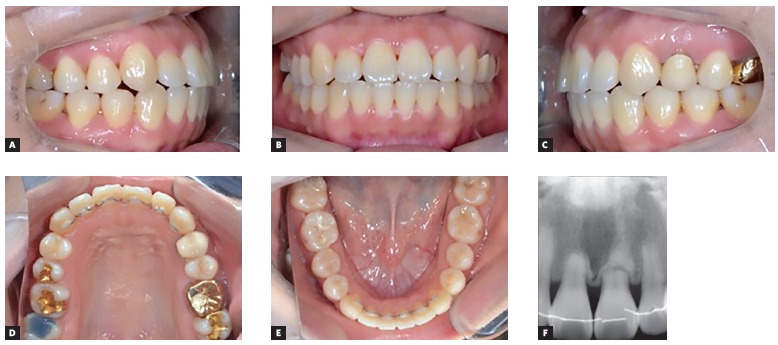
A-E) 3-year follow-up intraoral photographs and F) 2-year upper incisors periapical radiograph.
